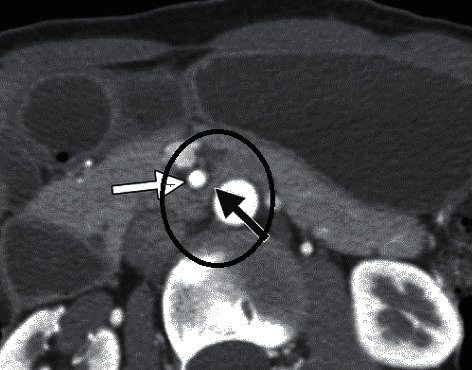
CT scan (axial view) with duodenal compression (black arrow) between the aorta and superior mesenteric artery (white arrows).
